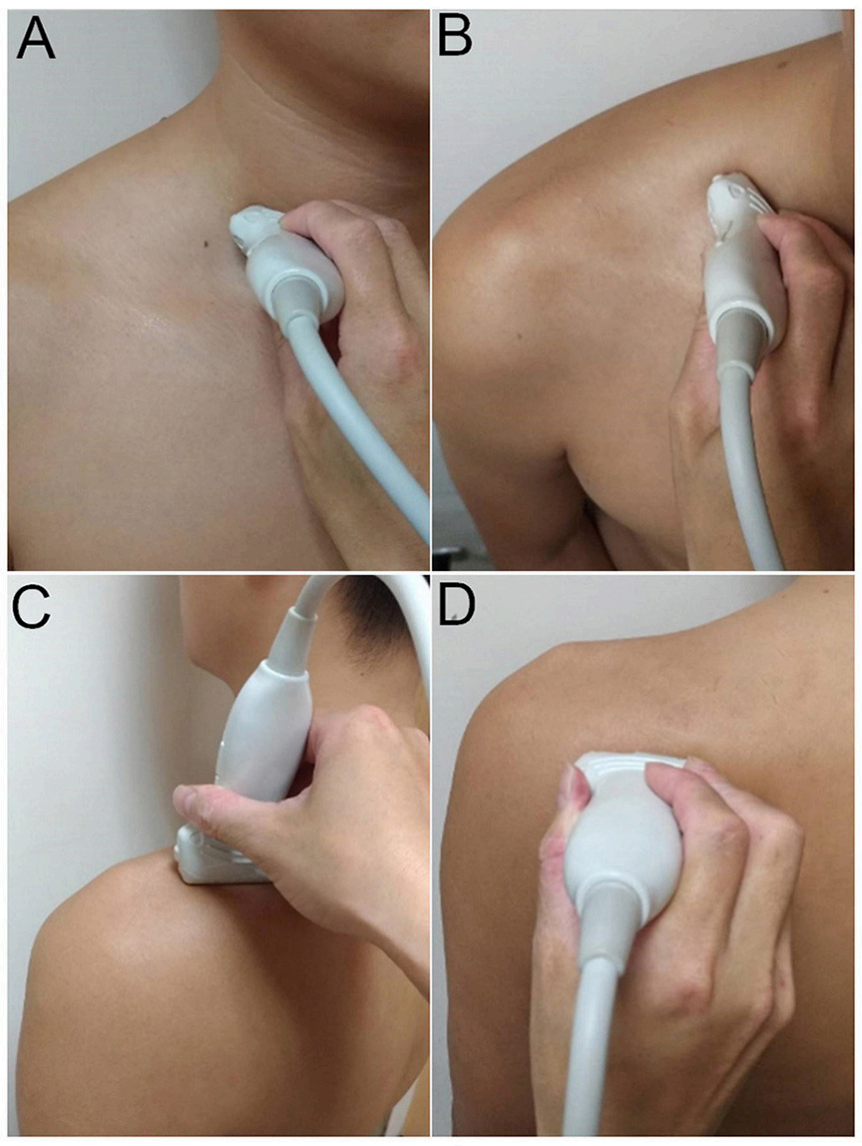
Placement of the ultrasound transducer on the participants for visualization of cervical nerve roots (A) and the suprascapular nerve at the supraclavicular region (B), inside the supraspinatus fossa (C), and at the spinoglenoid notch of the infraspinatus fossa (D).
